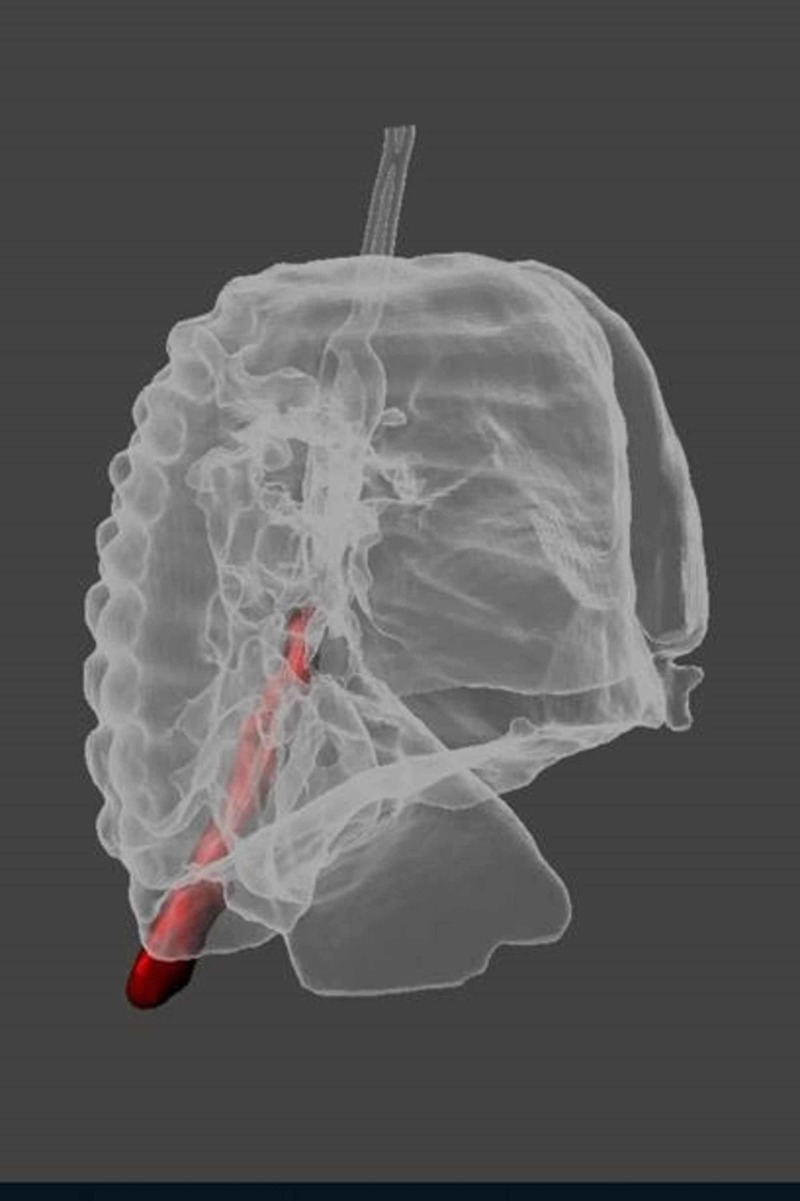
3D view of the chest CT - lateral projection Lateral projection of the 3D reconstruction of the chest CT demonstrating the position of the foreign body (red) prior to removal CT: computed tomography
